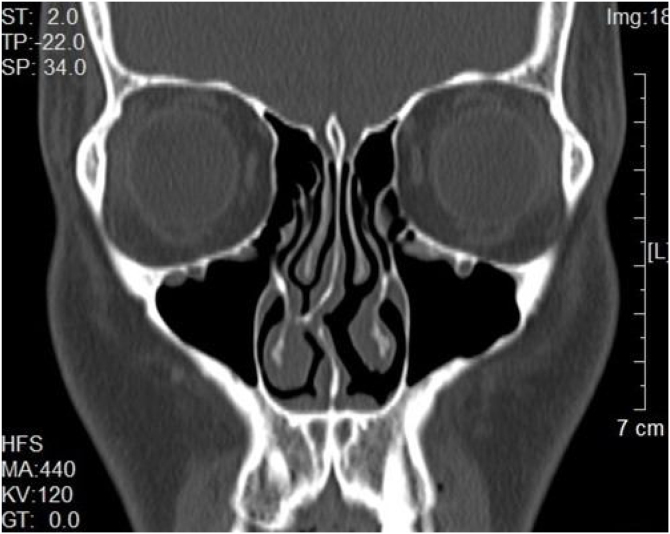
Preoperative CT imaging of the nasal septum with deviation to the right and compression of the ipsilateral inferior turbinate.
